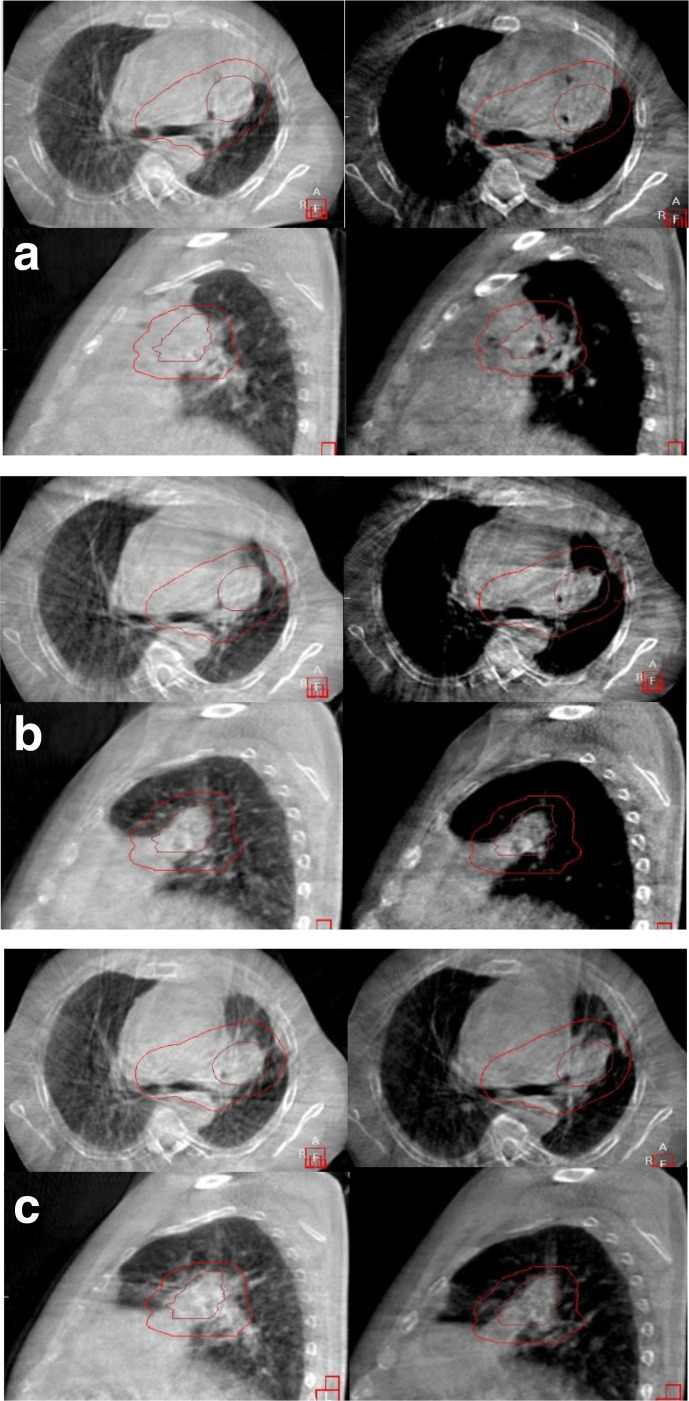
Development of the atelectasis during thoracic irradiation. (A) End of 04/2020: first day of irradiation – atelectasis of the left upper lobe can be seen on kilovoltage CBCT. (3.5/52.5 Gy; EQD2 (α/β 10): 3.9 Gy). (B) Early 05/2020: reaeration of the left upper lobe bronchus begins. Segmental bronchi are opening. The patient reports improvement in dyspnea (45.5/52.5 Gy; EQD2 (α/β 10): 51.19 Gy). (C) Mid 05/2020: on CBCT before the last fraction, almost complete resolution (52.5/52.5 Gy; EQD2 (α/β 10): 59.06 Gy). Lung function examination: 03/2020 Prior to Radiotherapy: FEV1 1.43l (48.1% predicted), VCmax 1.82l (44.0% predicted) Early 06/2020 After Radiotherapy FEV1 2.49l (82.6% predicted), VCmax 3.17l (76.6% predicted). CBCT, cone-beam CT.
